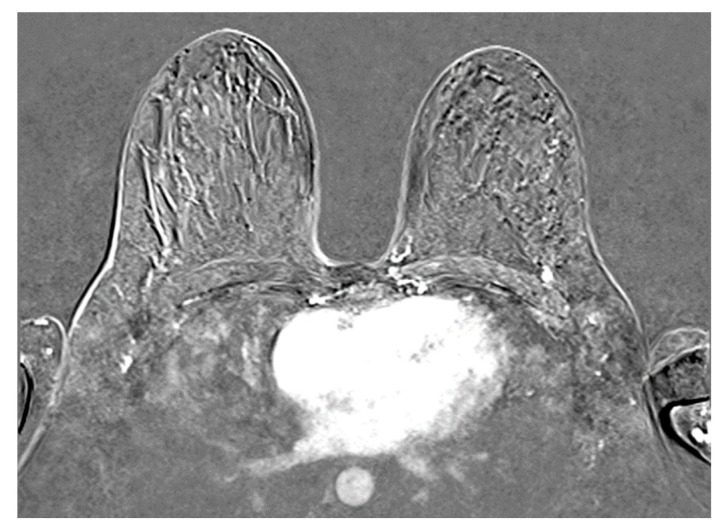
Post treatment control MRI (institutional patient data).
